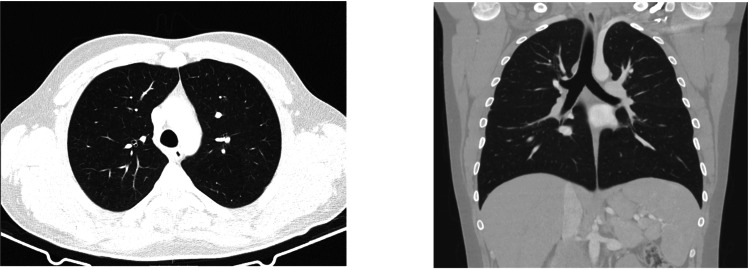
Thoracic CT scan on the ninth day of hospitalization. Left: axial view. Right: coronal view
